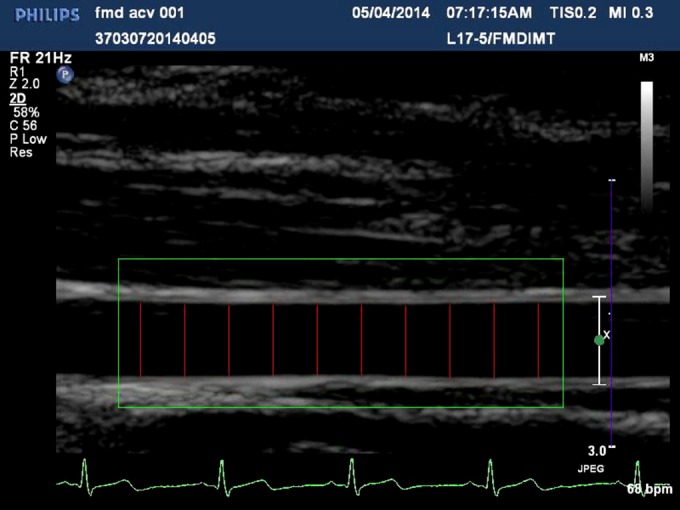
Sonogram of the brachial artery, 10 cm proximal from the elbow, green box determines the region of interest. Ten equidistant red lines were used to determine the frame by frame diameter difference over 180 seconds (15 seconds precuff release until 165 seconds).
